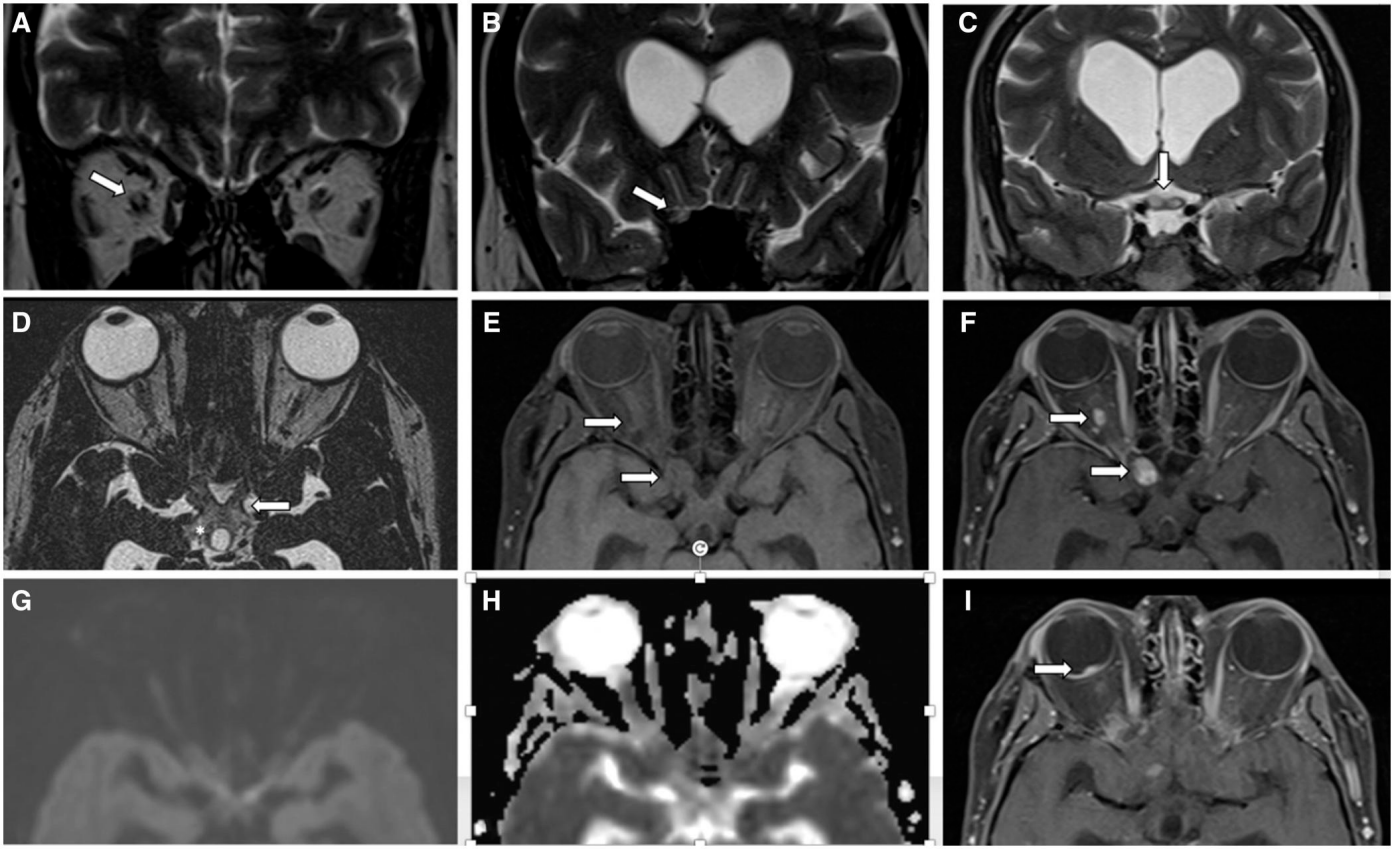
Haemangioblastomas of the optic nerve and retina. Axial (A) and coronal (B) T2-weighted MRI of the orbit show a hyperintense right optic nerve in intraorbital and intracanalicular segment (arrows), and coronal (C) and axial (D) T2-weighted MRI show hyperintense optic chiasm (arrow) with extension to optic radiation bilaterally (asterisk). Axial T1-weighted MR image (E) and contrast-enhanced T1-weighted MR image (F) show focal lesions with avid enhancement in the intraorbital and intracanalicular segment of the right optic nerve. No restriction in DWI and ADC map (F, G). Axial (E) contrast-enhanced T1-weighted MR image shows an enhancing nodular focal lesion on the optic disc (arrow).
